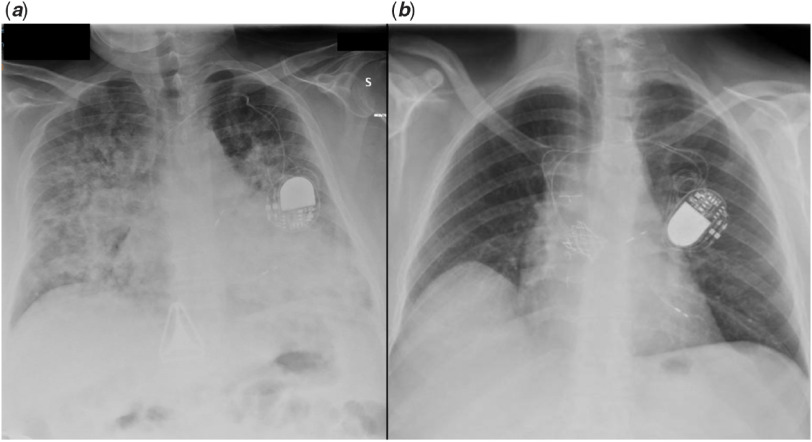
Chest X-ray, frontal view showing diffuse alveolar-interstitial infiltrates in case 2 (*a*) and normal lung fields in case 3 (*b*).
